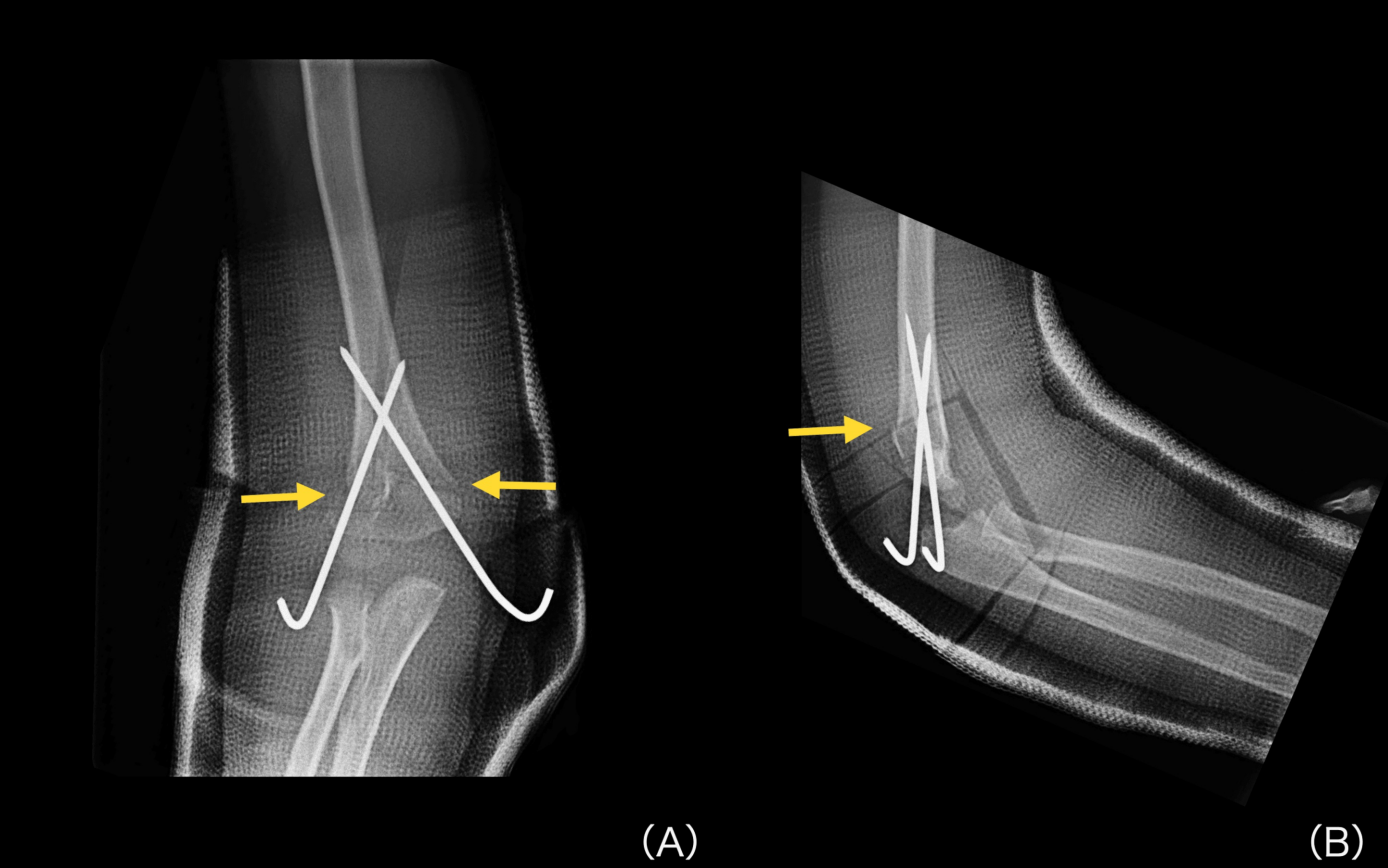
Immediate postoperative radiographs after percutaneous pinning of a supracondylar humerus fracture Immediate postoperative plain radiographs of the right elbow after percutaneous pinning, showing crossed Kirschner-wire fixation of the supracondylar humerus fracture: anteroposterior view (A) and lateral view (B). Yellow arrows indicate the fracture lines.
